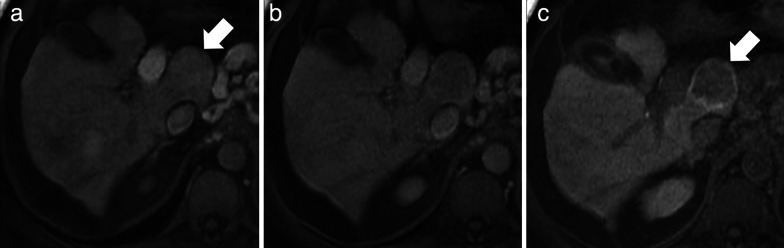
A 55-year-old man with HCV-related cirrhosis and multiple HCCs. Gadoxetate disodium-enhanced MRI shows an HCC mass in the caudate lobe with (a) arterial phase hyperenhancement in the arterial phase, (b) non-peripheral washout in the portal venous phase and (c) hypointensity in the hepatobiliary phase with peripheral hyperintensity (arrow), suggesting microvascular invasion
